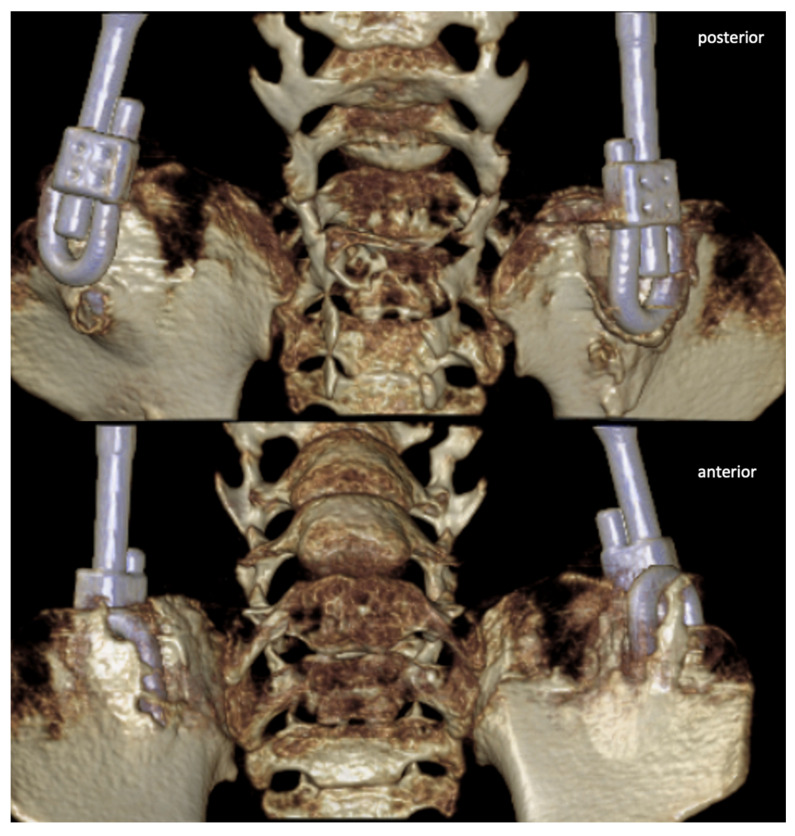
CT graphic 3D reconstruction showing heterotopic ossification around the S-hook. (Top) picture shows a posterior view, whilst the (bottom) picture depicts an anterior view.
